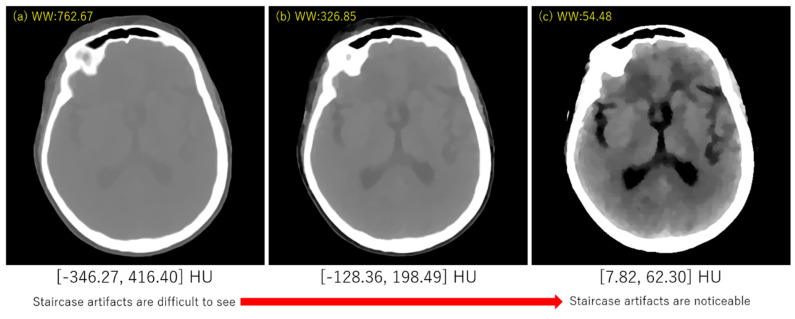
Demonstration of appearance of the staircase artifacts with various gray-scale ranges in displaying the brain CT image. (a) Window Width [−346.27, 416.40] HU, (b) Window Width [−128.36, 198.49] HU, (c) Window Width [7.82, 62.30] HU.
